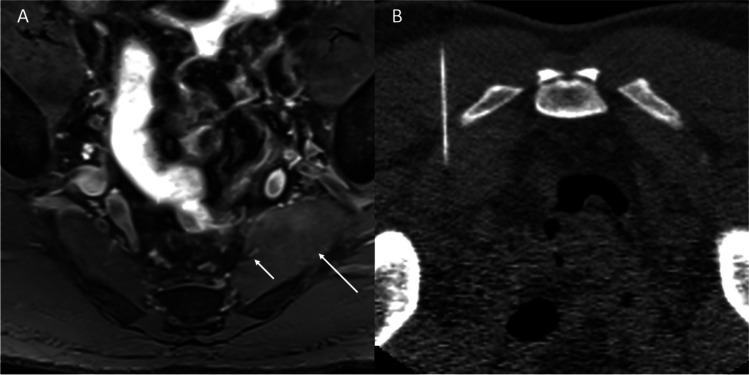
T2 SPAIR axial image (A) of the pelvis showing hypertrophy of the left piriformis muscle (arrow) in a male patient with left gluteal pain. The left S2 nerve (short arrow) has an intramuscular course within the medial edge of the left piriformis muscle. A CT-guided botox injection (B) of the enlarged left piriformis muscle was subsequently performed and provided satisfactory relief of symptoms
